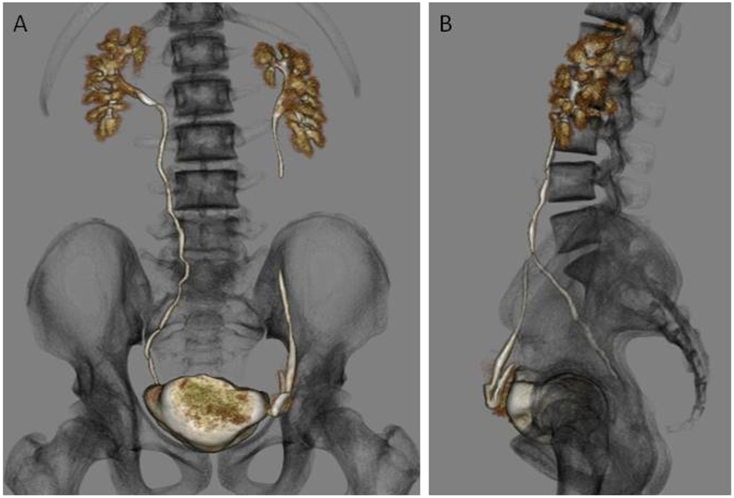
Postoperative drip infusion pyelography-computed tomography (DIP-CT). The left ureter reached the inguinal canal via the internal inguinal ring, turned over with a loop, and was connected to the bladder via the internal inguinal ring.
